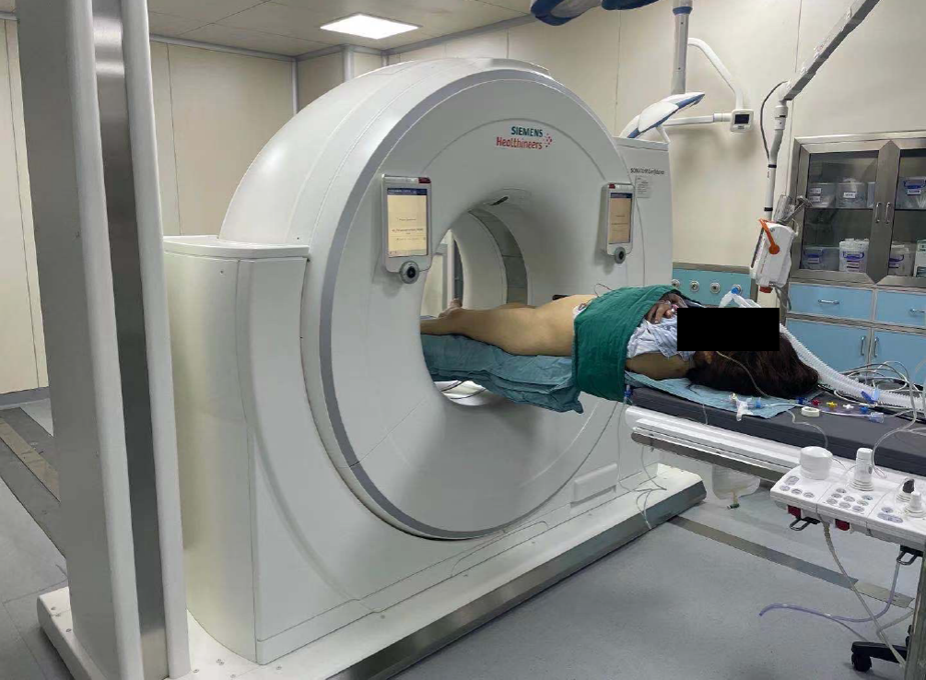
Standard posture of the patients with sacroiliac screw fixation assisted by intraoperative CT.
